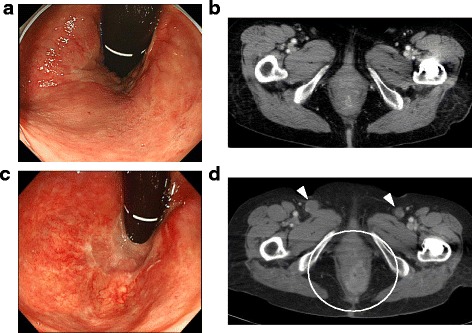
No specific target lesions were observed by rectal endoscopy (a) and CT (b). Endoscopy showed that anorectal mucous membranes had become rough (c). CT revealed thickening of the anorectal wall (circle) and bilateral inguinal lymph node metastasis (arrow head) (d)
